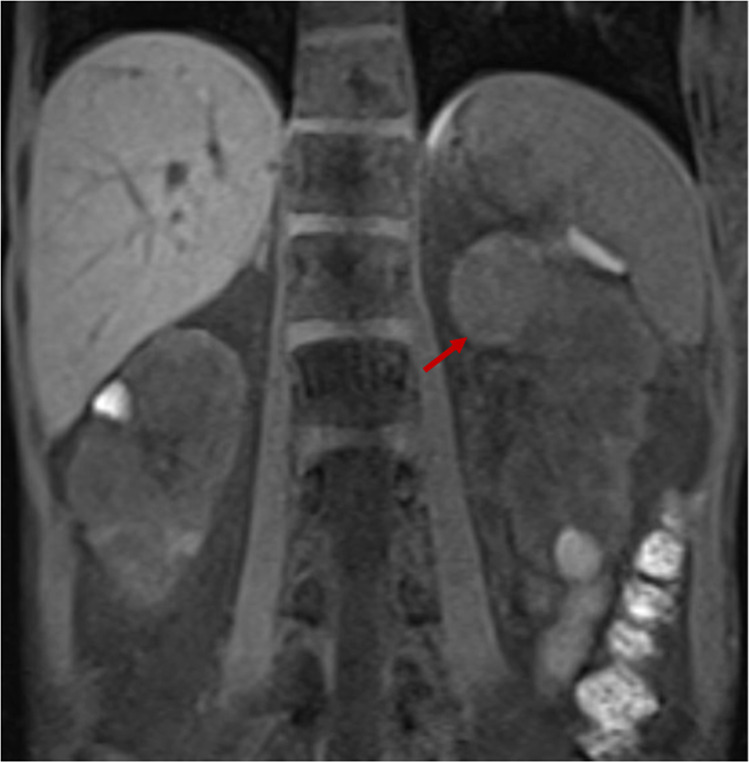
Patient 2 imaging. MRI of kidneys showing bilateral neoplasms with the largest (arrow) in the medial aspect of the upper pole of the left kidney.
